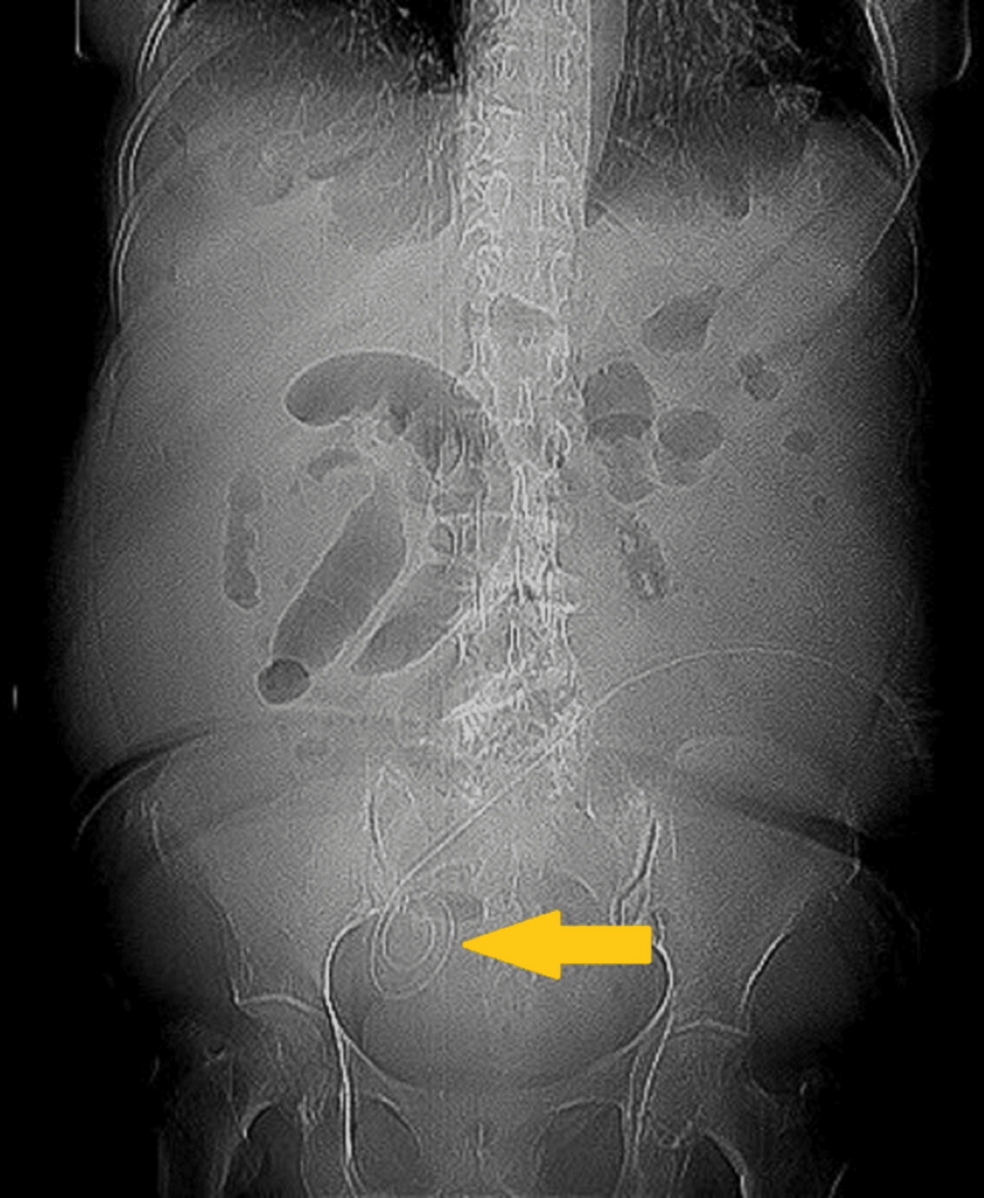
Abdominal X-ray The yellow arrow shows the location of the Tenckhoff catheter in the right iliac fossa, not twisted or bent.
